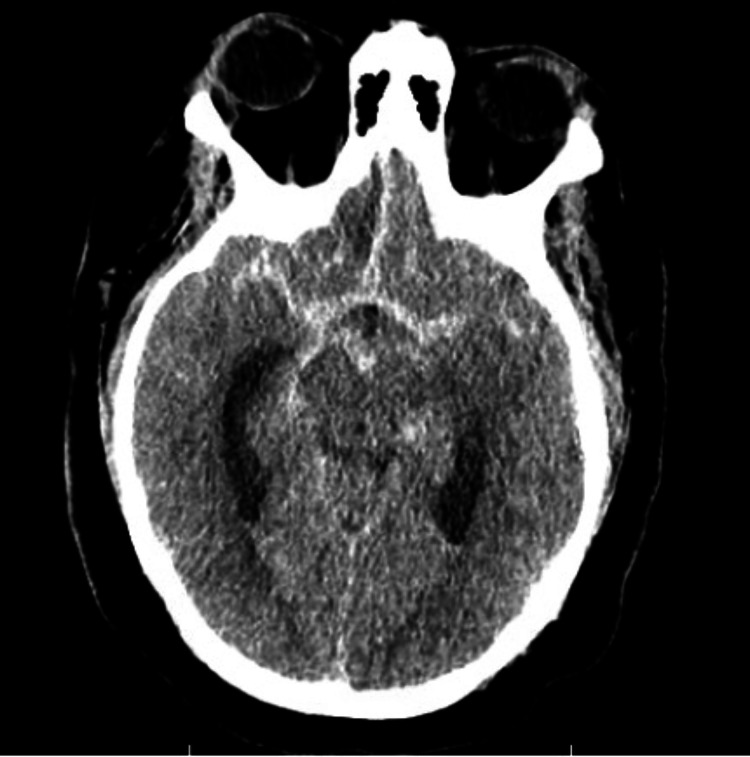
Non-contrast computed tomography scan with diffuse cisternal subarachnoid hemorrhage extending into the interhemispheric fissure with associated obstructive hydrocephalus
